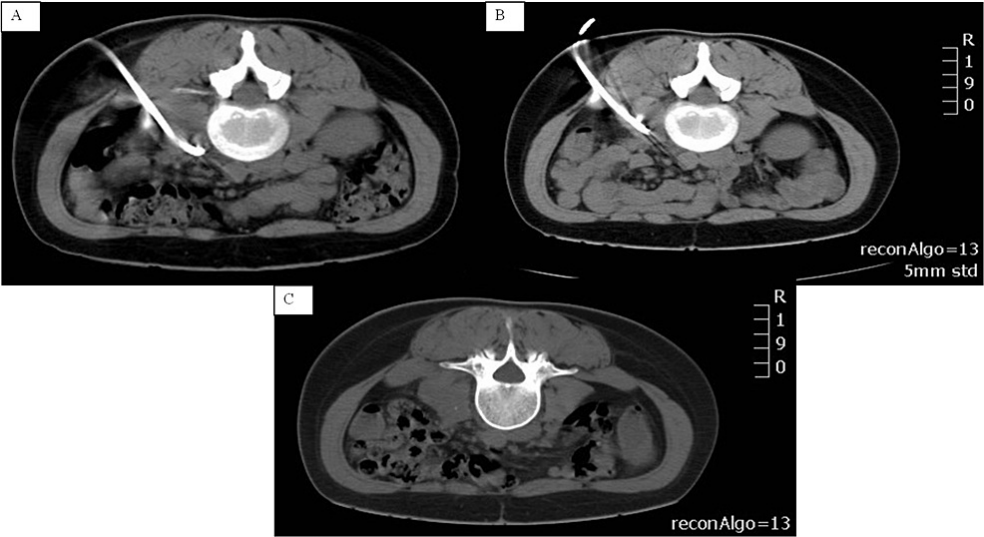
Percutaneous drainage of the psoas abscess under radiological guidance A: CT-guided pigtail catheter insertion for the drainage of the psoas abscess; B: pigtail catheter removal after complete drainage of the purulent fluid; C: the significant resolution of the psoas abscess achieved through percutaneous drainage.
